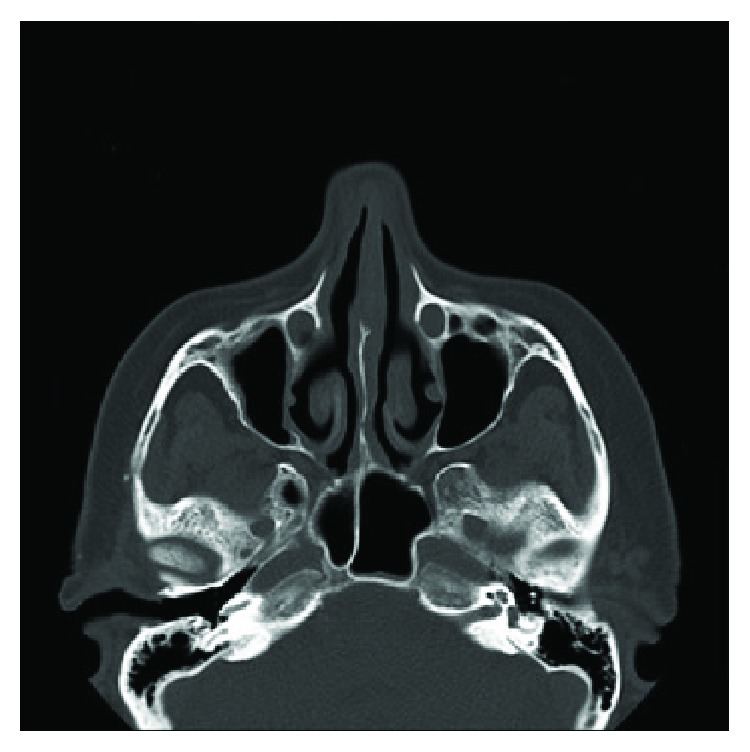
Postoperative axial CT scan.
